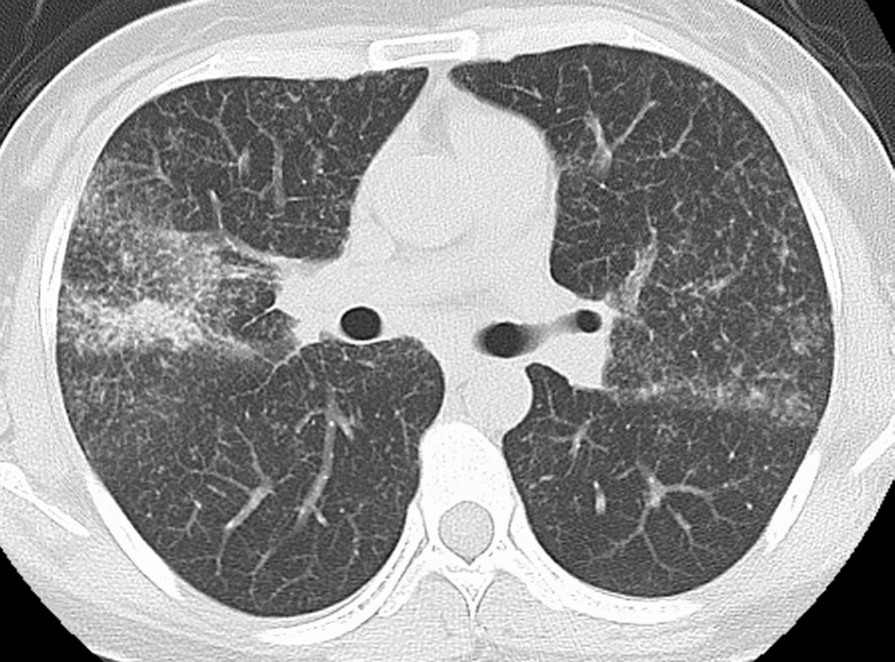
CT scan showing multiple nodular lung lesions and mediastinal and hilar lymphadenopathies
